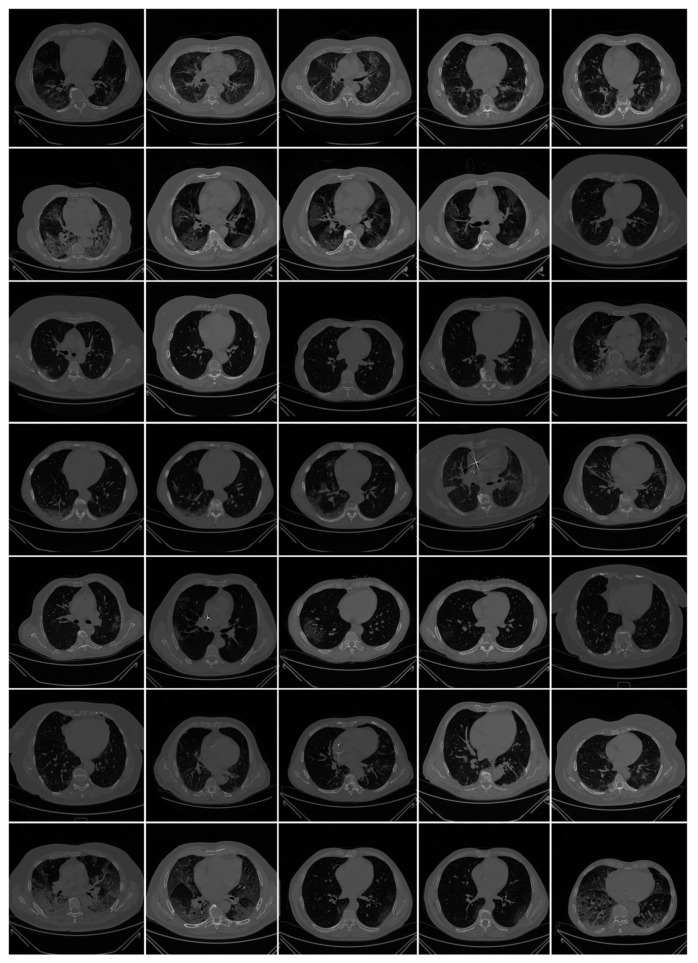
Raw lung COVID-19 CT scans taken from different patients in the database.
